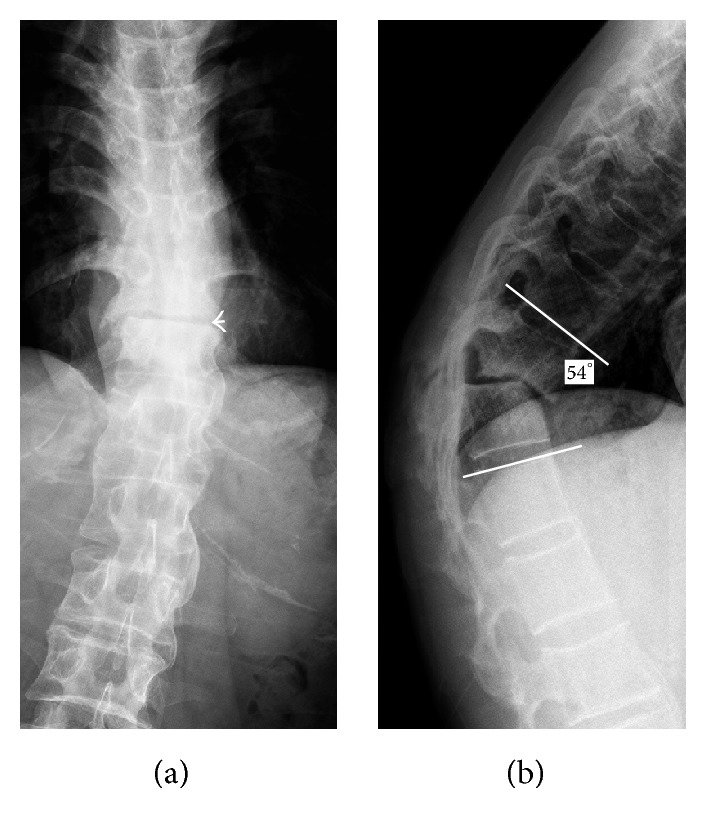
AP and lateral radiographs of spinal pseudarthrosis in AS. Radiographs showed a rigid spine with pseudarthrosis (a, arrow) at the T11-T12 level and a local kyphosis of 54° (b).
